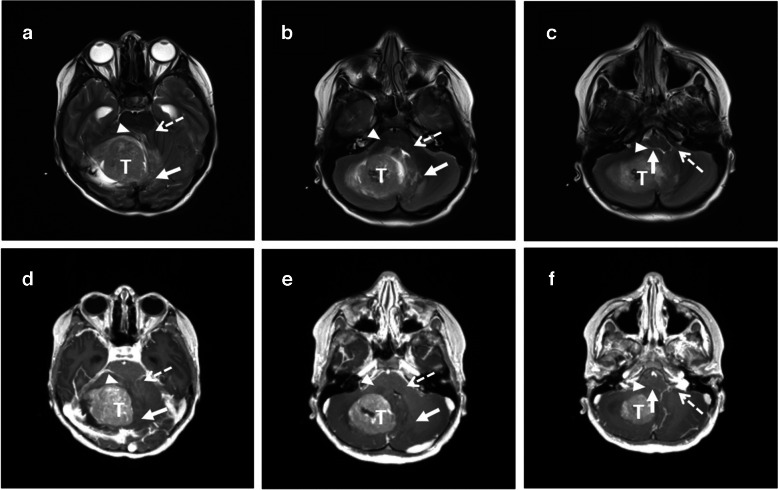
Axial T2 (a–c) and post-contrast T1 (d–f) magnetic resonance images in a 6-year-old girl (patient 7) with a posterior fossa tumour. Slices have been obtained at the level of the superior (a, d), middle (b, e), and inferior (c, f) cerebellar peduncles. a, d Compression of the vermis has a score of 4 (*arrow*), of the right superior cerebellar peduncle a score of 4 (*arrowhead*), and of the left superior cerebellar peduncle a score of 2 (*broken arrow*). b, e Compression of the region of the left dentate nucleus has a score of 3 (*arrow*), of the right middle cerebellar peduncle a score of 4 (*arrowhead*), and of the left middle cerebellar peduncle a score of 2 (*broken arrow*). c, f Compression of the medulla oblongata has a score of 3 (*arrow*), of the right inferior cerebellar peduncle a score of 3 (*arrowhead*), and of the left inferior cerebellar peduncle a score of 2 (*broken arrow*)*.* Scoring references were provided by the most experienced radiologist (R.D.). *T* posterior fossa tumour
